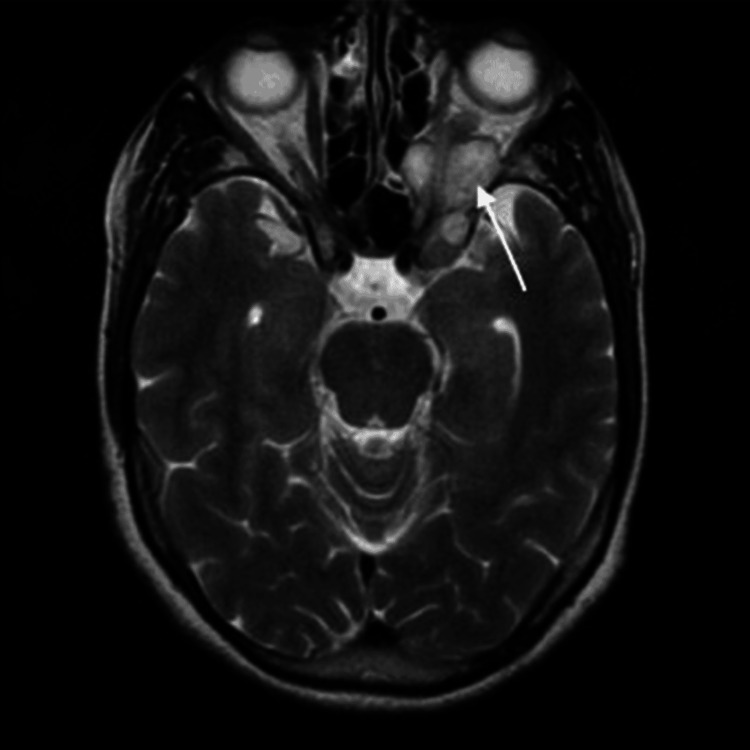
MRI scan of brain and orbit showing smooth enhancing lesion in retrobulbar region Arrow shows hyperintense lesion along the optic nerve (arrow), extending to middle cranial fossa
